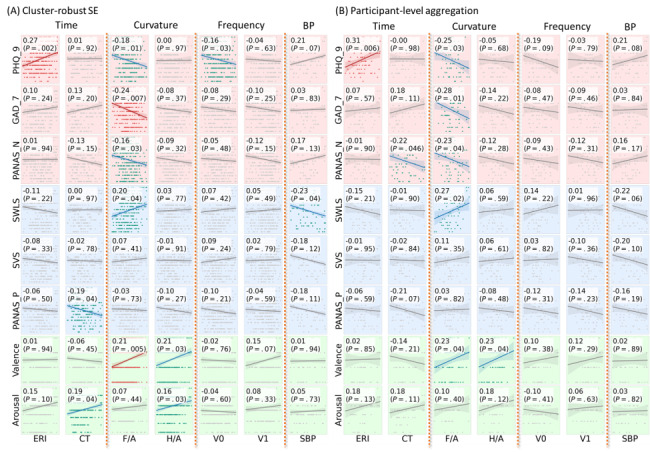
Univariate relationships between each independent and dependent variable. Green markers and regression lines denote *P* value less than .05 and red markers denote *P* value less than the Bonferroni corrected criteria .05/9=.0056. CT: crest time; ERI: estimated reflection index; GAD-7: Generalized Anxiety Disorder-7; PANAS: positive and negative affect schedule; PANAS-N: negative affect; PANAS-P: positive affect; PHQ-9: Patient Health Questionnaire-9; SBP: systolic blood pressure; SVS: Subjective Vitality Scale; SWLS: Satisfaction with Life Scale.
